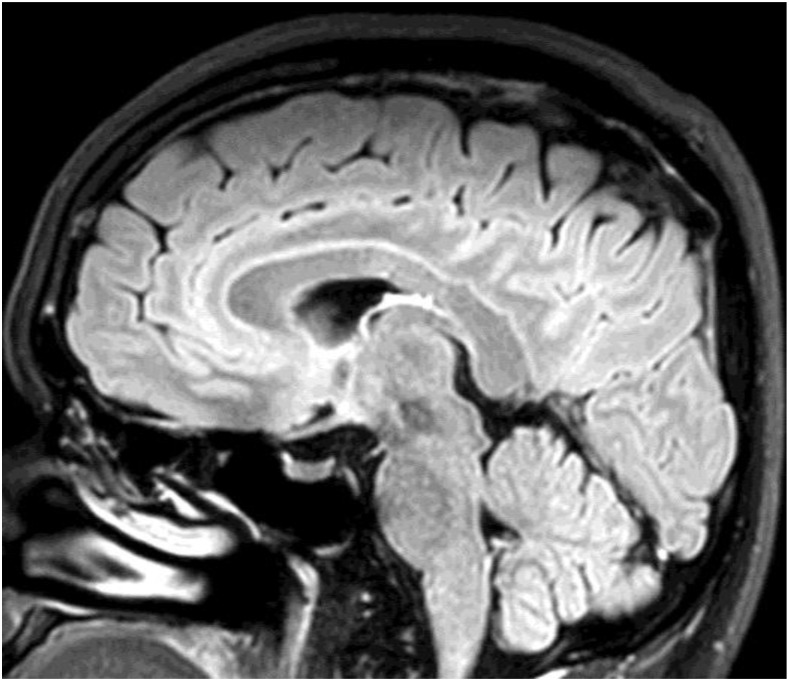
Sagittal fluid-attenuated inversion recovery magnetic resonance imaging of the brain at a 6-month follow-up showing complete resolution of the lesion.
